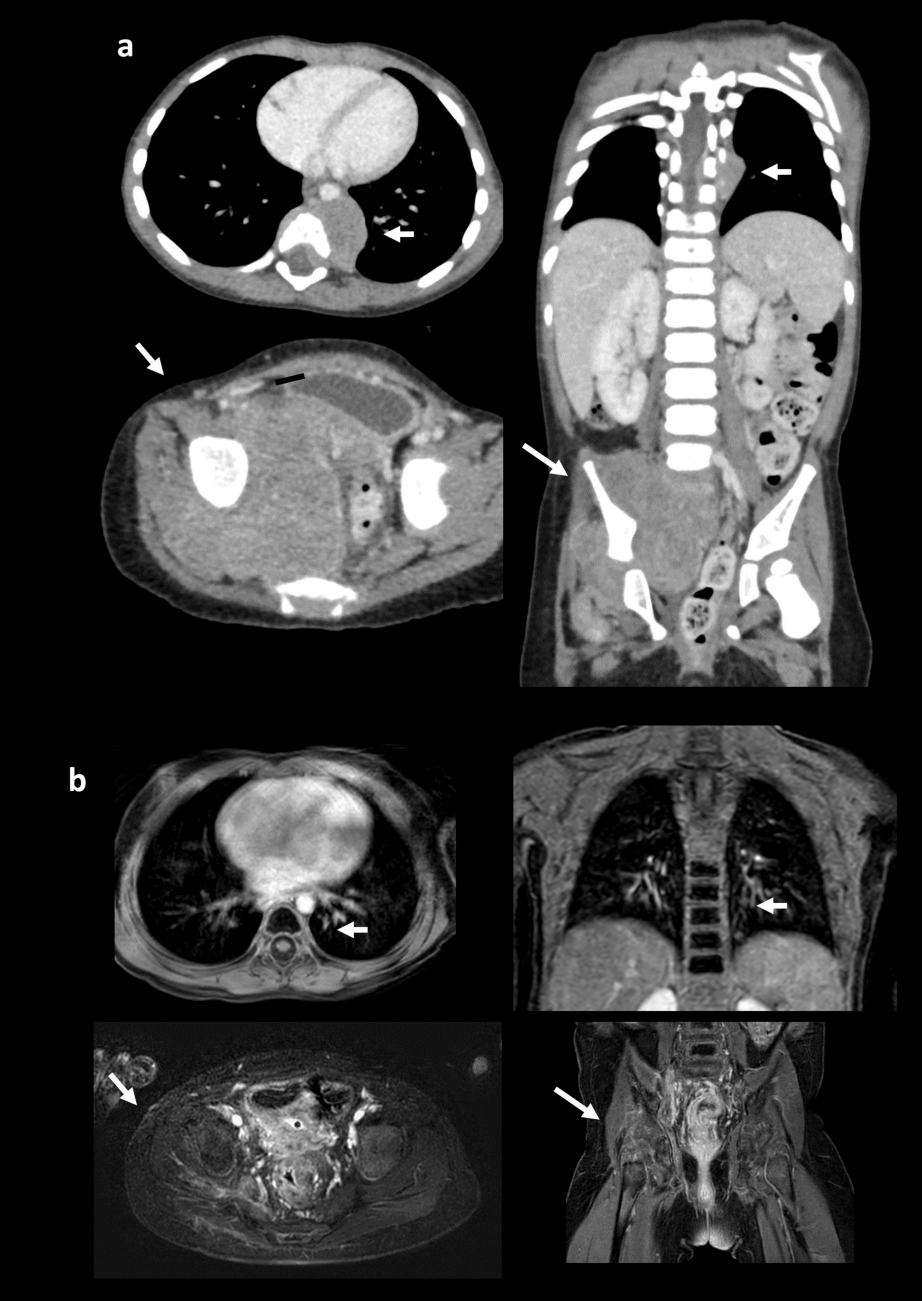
A bulky tumor of the pelvis and parathoracic vertebral metastasis a: Computed tomography revealed a bulky tumor in the right pelvis extending into the pelvic bone and spinal cord, and another tumor located at the seventh to eighth parathoracic vertebrae. b: Magnetic resonance imaging 12 months after diagnosis showed shrinkage of the metastatic tumor in the thoracic spine as well as the primary pelvic tumor.
